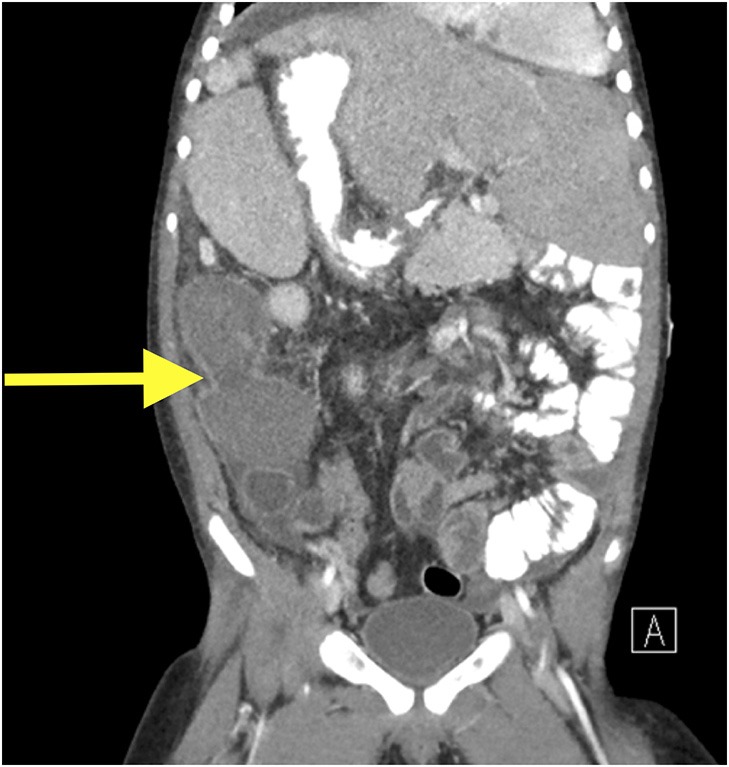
Coronal CT scan demonstrating a right intra-abdominal rim enhancing lesion.
